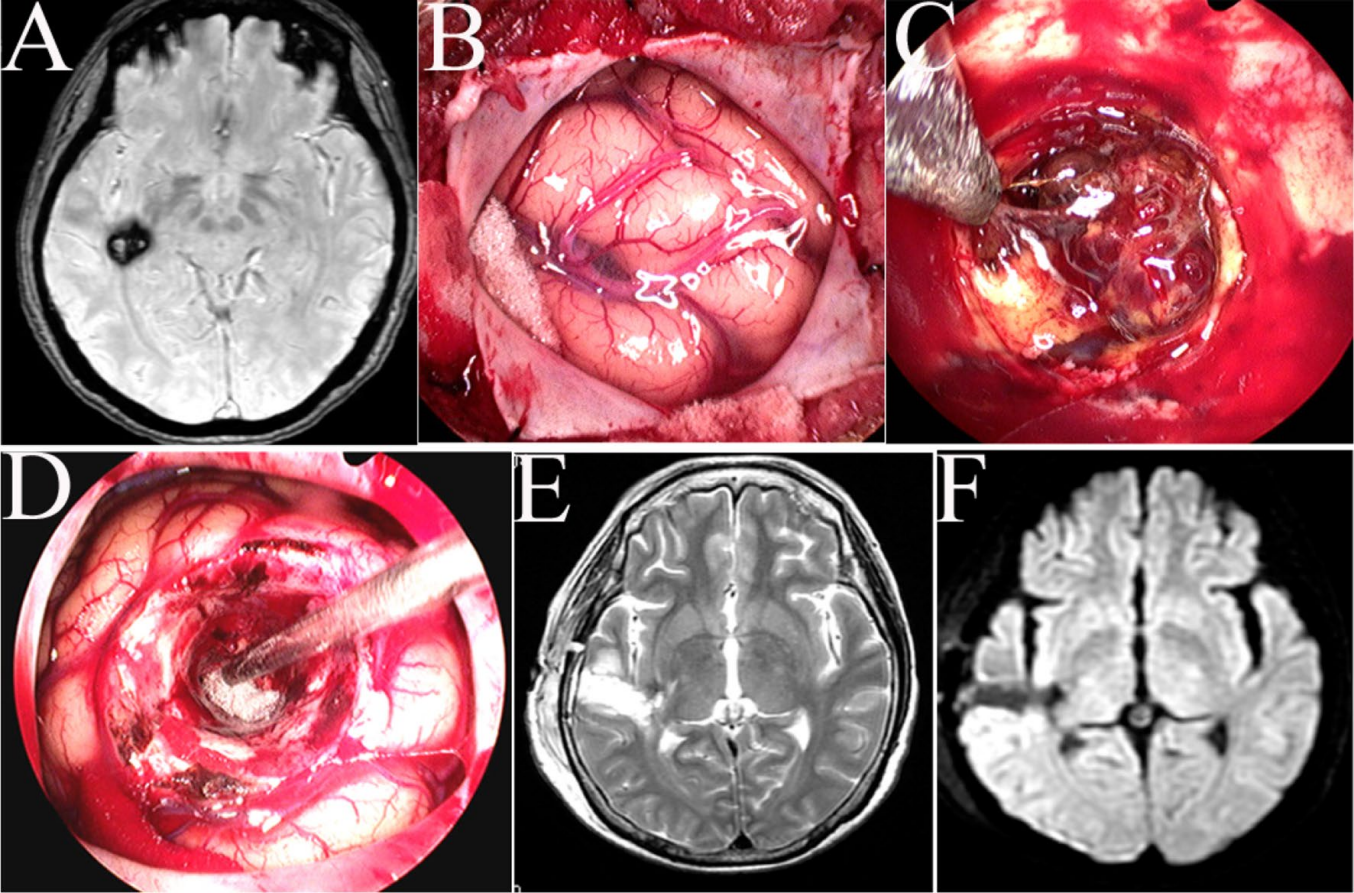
A patient with a small cavernous hemangioma in the right medial temporal lobe was treated under neuroendoscopy. (A) Preoperative magnetic resonance imaging (MRI) showed a small cavernous hemangioma in the right temporal lobe. (B) Cross incision of the dura matter to expose the temporal lobe. (C) The cavernous hemangioma was removed under direct visualization by neuroendoscopy. (D) The cavity of the cavernous hemangioma was checked. (E,F) T2-weighted axial and SWAN postoperative MRI showed complete removal of the cavernous hemangioma.
